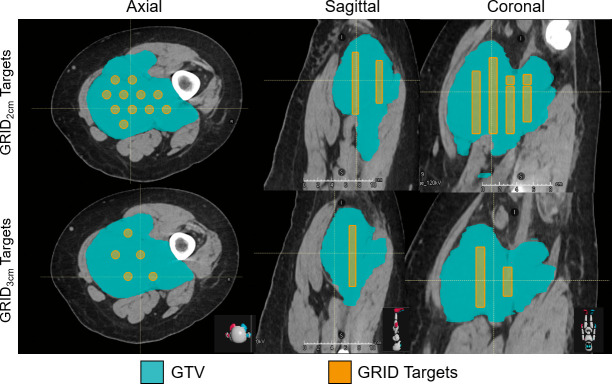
GRID target (orange) orientation with 2-cm (top) and 3-cm (bottom) spacing overlaid on CT in axial, coronal, and sagittal planes. The GTV is shown in cyan. Abbreviations: CT, computed tomography; GRID, spatially fractionated radiation therapy; GTV, gross tumor volume.
